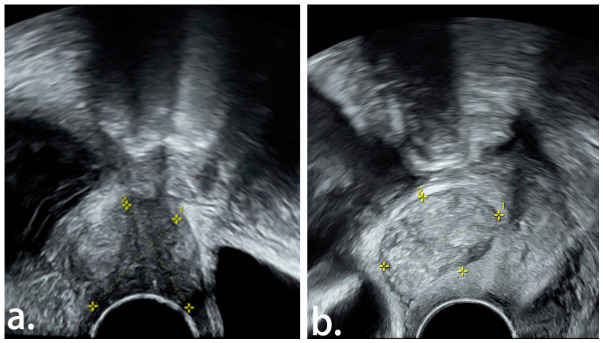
Comparative abdominal TVUS images of cervical myoma and early cervical cancer. (a) A moderate-echo solid tumor (2.79 cm × 2.77 cm) was detected in the cervix, presenting with irregular morphology, partially ill-defined borders, and heterogeneous echo; pathologically confirmed as FIGO Stage IB2 cervical cancer after surgery. (b) A moderate-echo tumor (3.07 cm × 2.06 cm) was identified in the cervix, with well-defined borders and shallow lobulation; pathologically confirmed as a cervical myoma after surgery. Yellow cross calipers, numbered labels, and dashed lines in the figure denote the ultrasound measurement markers, which are used to measure the tumor’s maximum diameter (1) and anteroposterior diameter (2) and delineate the lesion boundaries.
